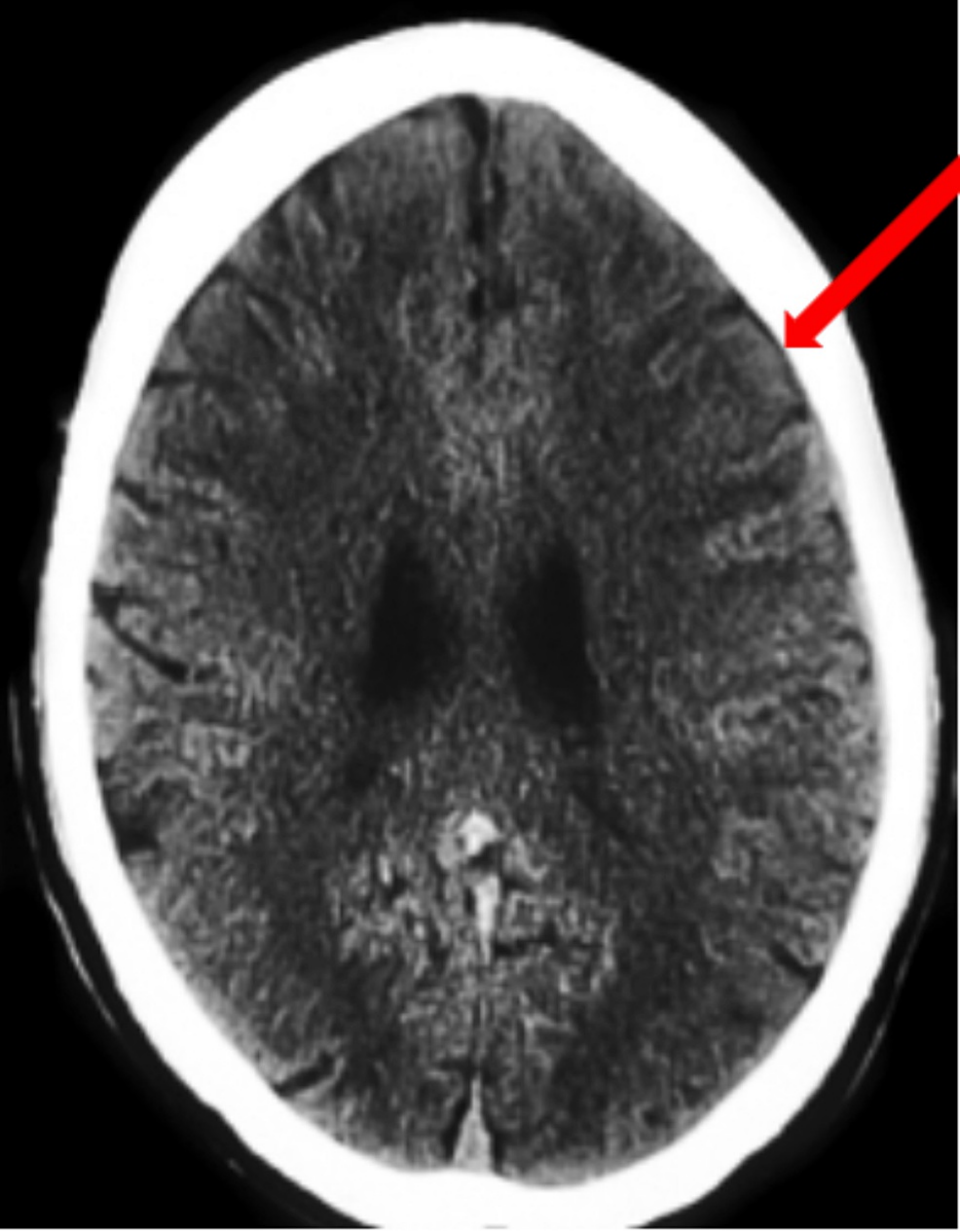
Final CT scan displaying final hematoma volume of 10 ml and less than 2 mm of midline shift
